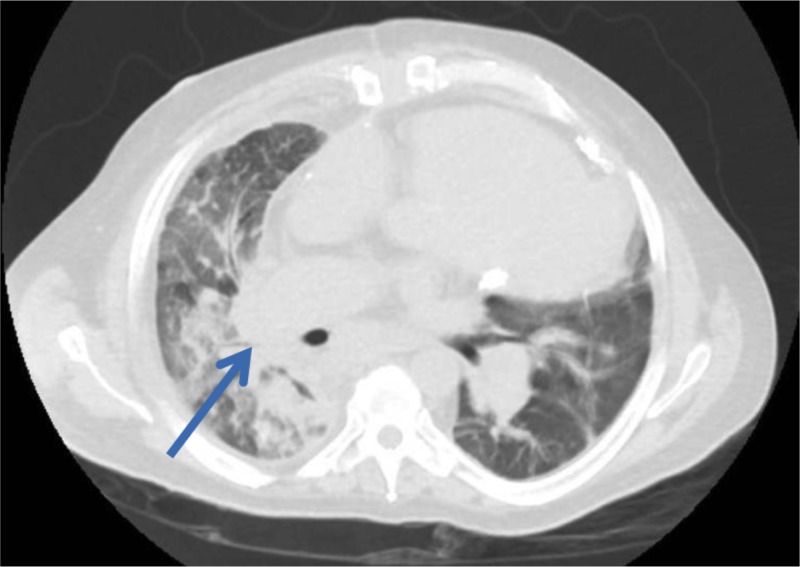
Computed tomography (CT) scan of the chest (lung window) showing total occlusion of right middle and lower lobe bronchus
